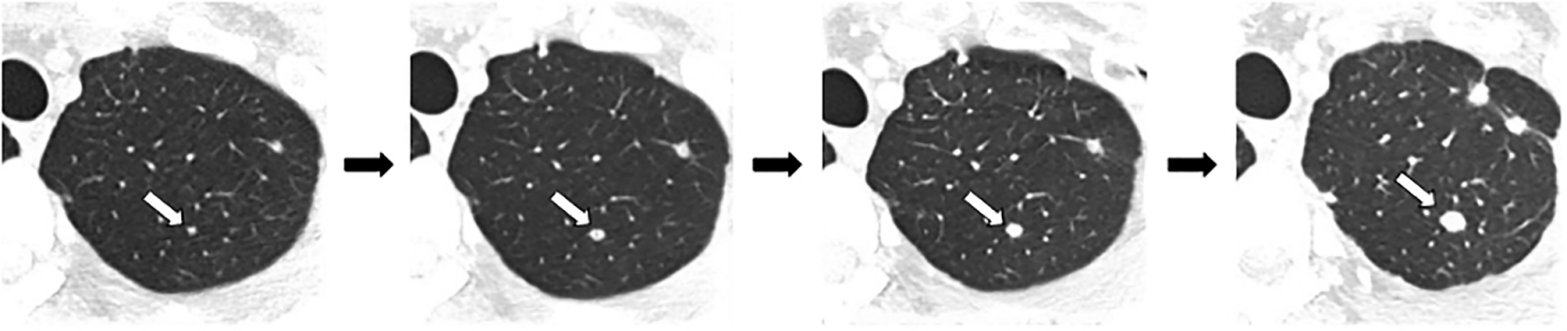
The process of nodule volume increasing on CT.
